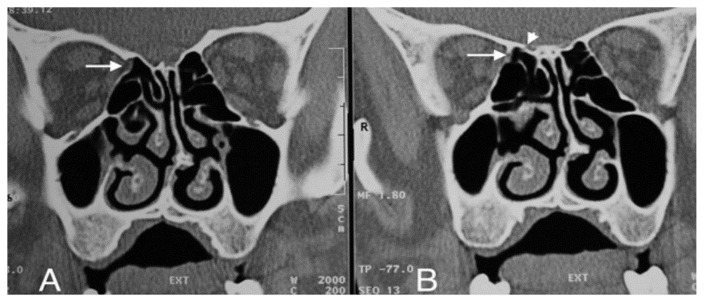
A- AEF-associated orbital fat tissue (white arrow) showing herniation from the right AEF level into the ethmoid sinus on the CT image. B- The orbital end of the ethmoidal canal (white arrow) and the cranial end (short white arrowhead) in the 3 mm posterior section.
